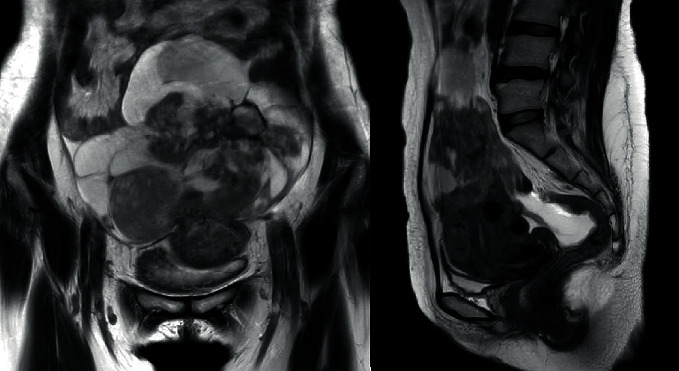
Magnetic resonance imaging was performed 3.5 years after uterine artery embolization. The leiomyomas were stable, and a new uterine mass was noted.
